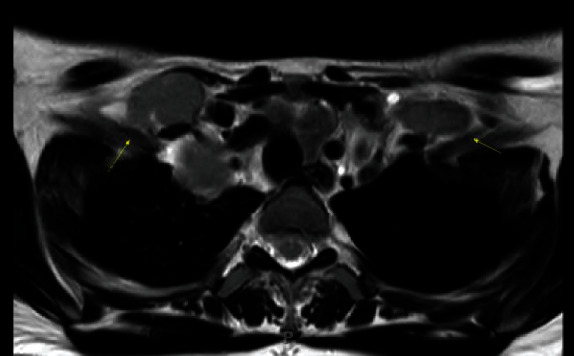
T2-weighted sequence: bilateral supraclavicular lymphadenopathy.
